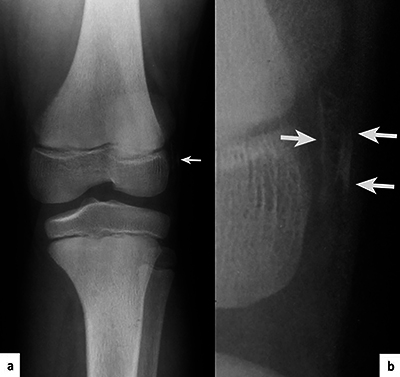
Thin linear ossifications of the soft tissues (arrows) on the lateral side of the lateral condyle, parallel to the skin, visible on the knee X-ray (a) and the magnified view (b).
